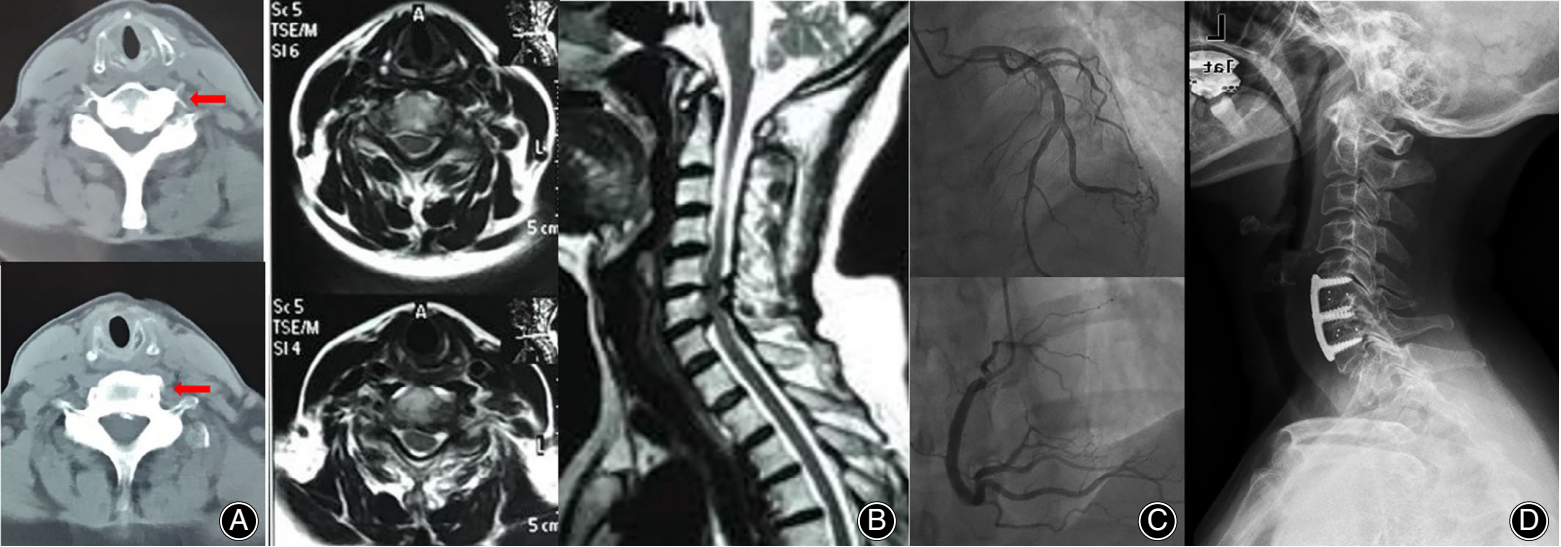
A case of a 47‐year‐old woman. MRI images show two segments (C_5–6_ and C_6–7_) of cervical disc hernia and neuroforaminal stenosis; the Luschka's joint osteophyte located at C_6–7_ level (A, B). Coronary artery angiography showed no artery stenosis (C). Anterior cervical discectomy and fusion (C_5–7_) surgery with resection of Luschka's joint osteophyte (D).
